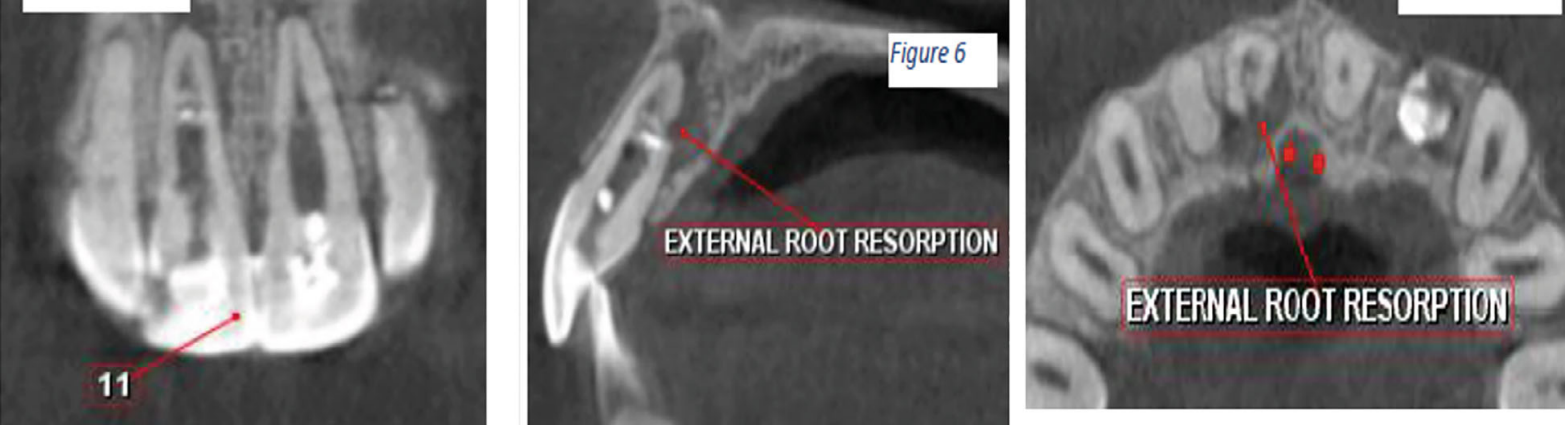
CBCT images showing coronal, sagittal and axial view of Tooth Number 11 with external root resorption.
